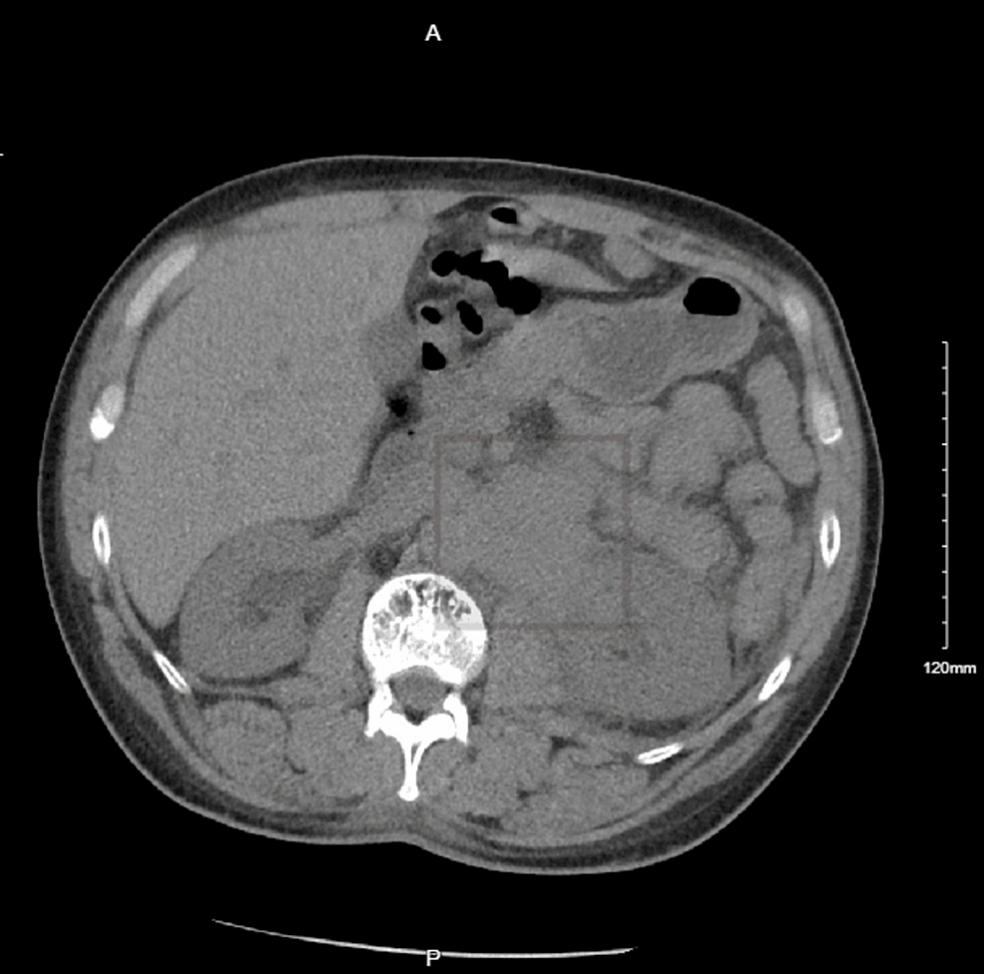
CT of the abdomen and pelvis without contrast showing confluent mass adjacent to the left side of the aorta at the level of the left kidney, poorly evaluated without intravenous contrast. CT: computed tomography
